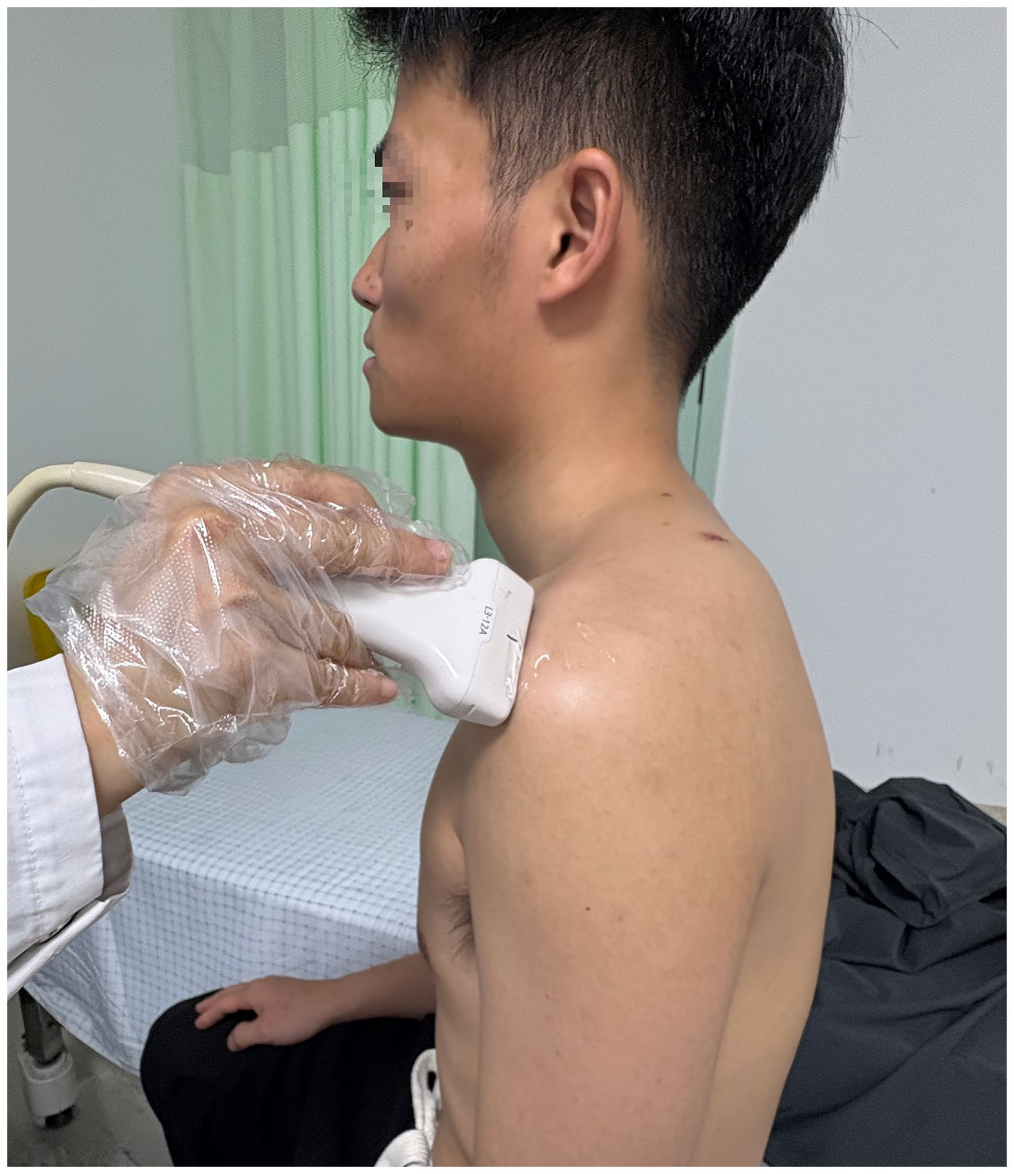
Participants’ standardized position for ultrasound assessment.
